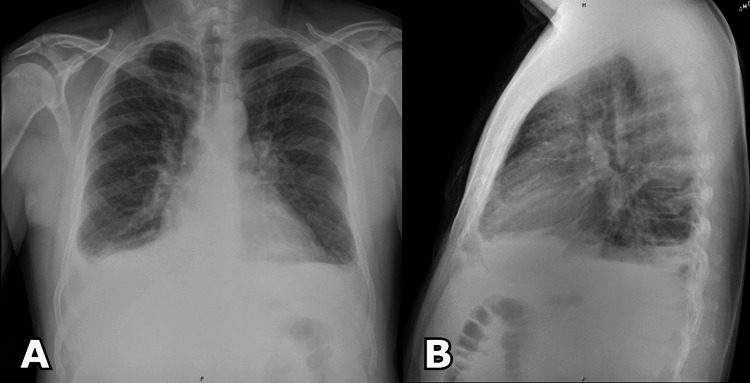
Anterior-posterior (A) and lateral (B) chest X-rays demonstrating pulmonary vascular congestion, cardiomegaly, and bilateral pleural effusions.
